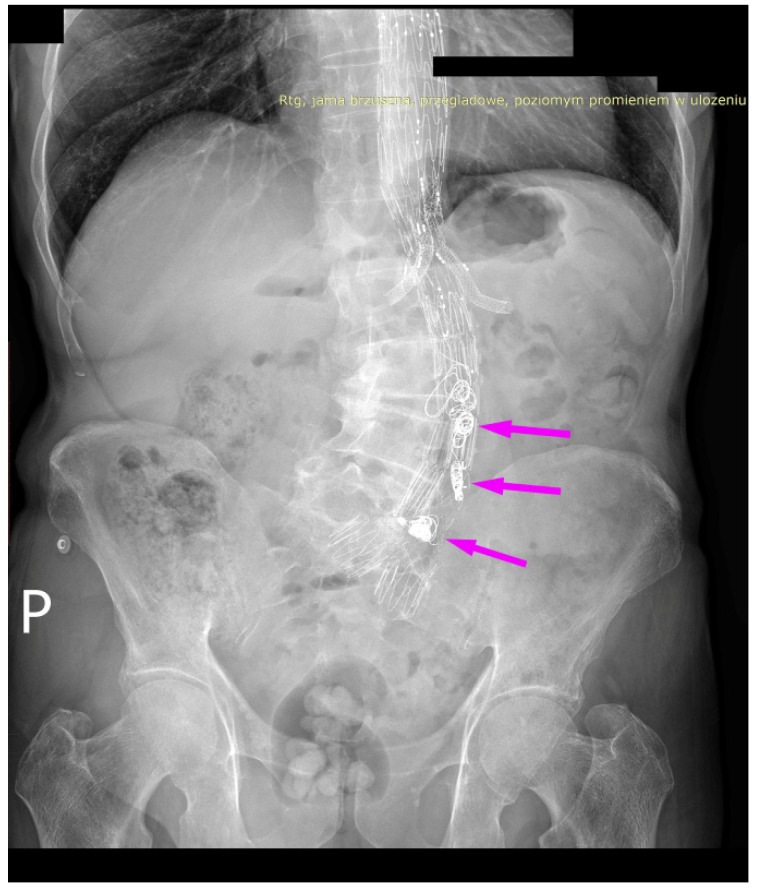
Thoracoabdominal branched stent-graft in the lumen of aorta and visceral arteries. Embolization coils are marked with arrows. Abdominal X-ray.
